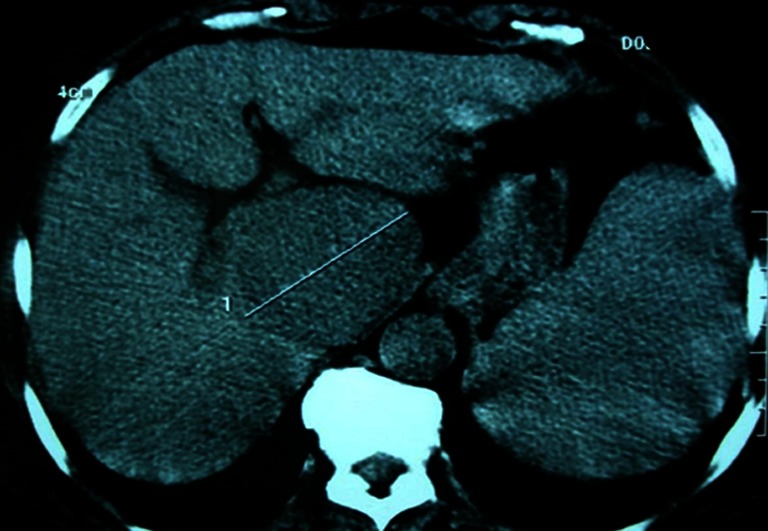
Abdominal CT scan without contrast showing a hypo-dense mass with regular contours, measuring 10 × 7 cm at the expense of the caudate lobe of the liver
